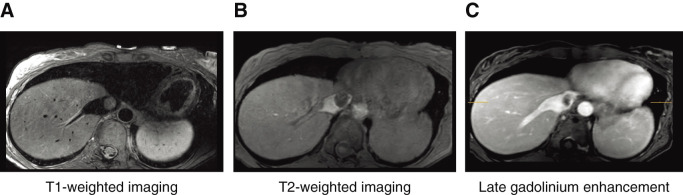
Fig. 3 (A) T1-weighted imaging showed a low signal with no fat components. (B) T2-weighted imaging showed a slightly heterogeneous, mildly high signal. (C) A faint enhancement effect was observed, predominantly at the margins.
